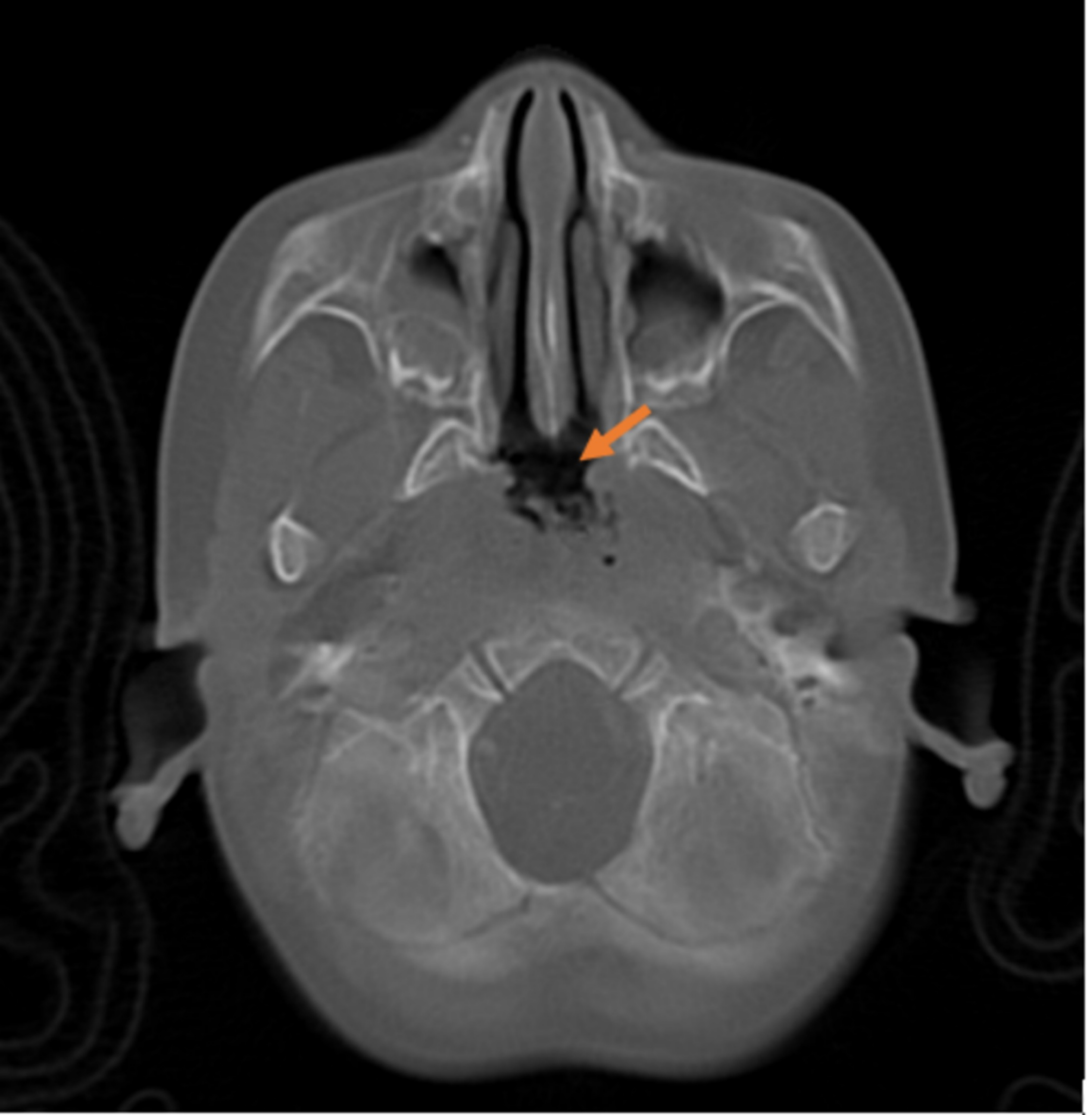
CT scan of nasopharynx two weeks following adenoidectomy Mild edema and mucus observed within the nasopharyngeal area (orange arrow). Additional views revealed normal bone in the skull base.
